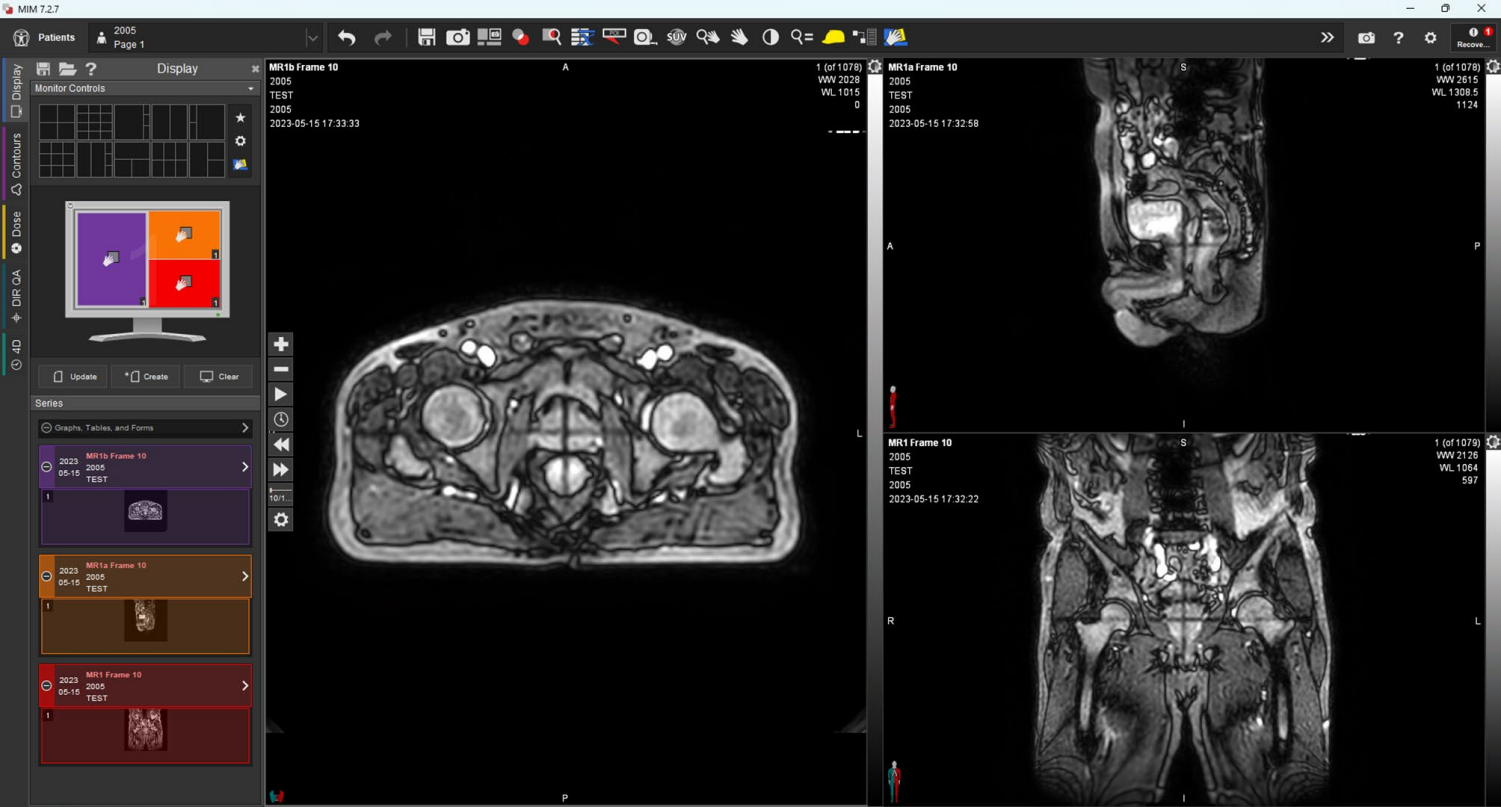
Converted 2D cine MR images acquired during MR-guided radiation treatment of a prostate cancer patient displayed in MIM software.
